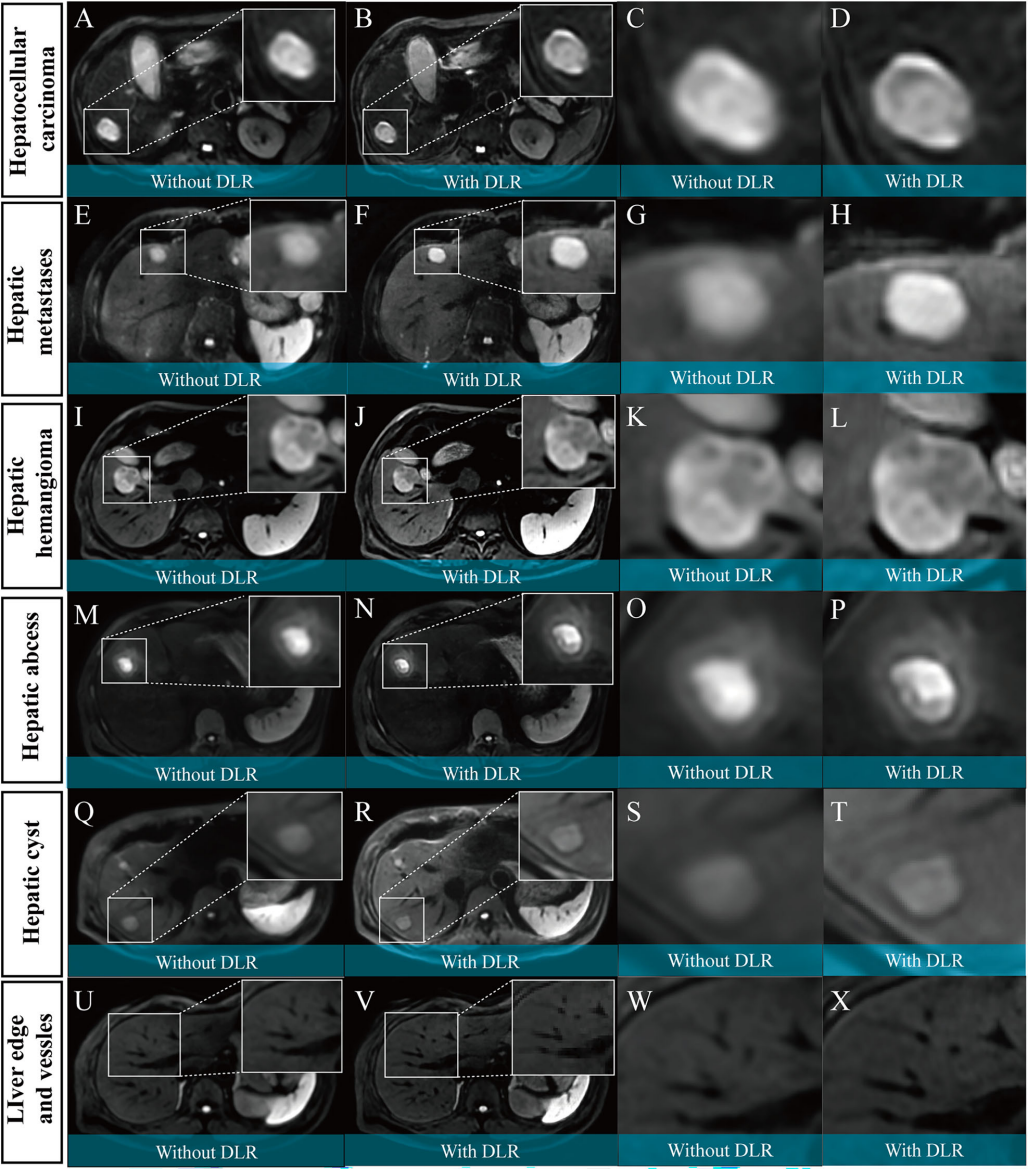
DWI images of Liver Lesions with and without DLR. The first and second columns show lesions without DLR (A, E, I, M, Q, U) and with DLR (B, F, J, N, R, V). The third and fourth columns are magnified views of the corresponding images. Lesions include hepatocellular carcinoma (A–D), hepatic metastases (E–H), hepatic hemangioma (I–L), hepatic abscess (M–P), and hepatic cyst (Q–T). The last row (U–X) shows the liver edge and vessels. The use of DLR demonstrates improved lesion visualization, enhanced edge sharpness, and better vessel clarity compared to conventional DWI
